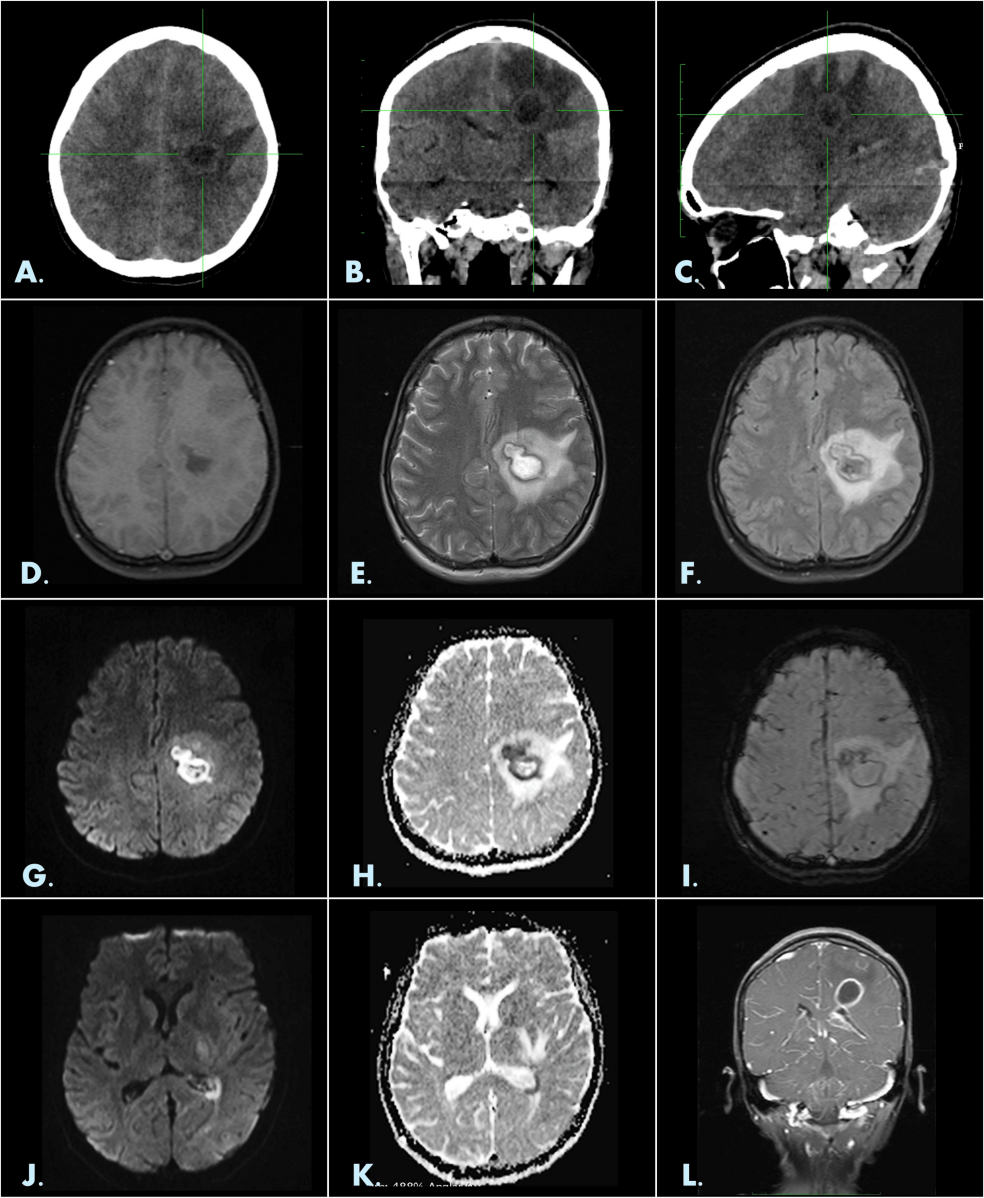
Imaging characteristics of the lesion in the left cerebral hemisphere: CT and MRI findings. Initial non-enhanced CT of the brain showing a 22 × 16 × 18 mm thin-walled lesion with central hypodensity in the left centrum semiovale and corona radiata, accompanied by perilesional edema. Axial (A), coronal (B), and sagittal (C) sections are displayed. MRI images reveal the lesion as hypointense on T1-weighted images (D), hyperintense on T2-weighted images (E), and heterogeneously hyperintense on FLAIR sequences (F). Diffusion restriction is shown (G and H). A dual rim sign is observed on susceptibility-weighted images (I). Additionally, diffusion restriction within the left lateral ventricle suggests rupture into the ventricle (J and K). Post-contrast MRI showed smooth, thin ring enhancement of the lesion with mild enhancement along the ependymal lining of the left lateral ventricle (L). CT, computed tomography; MRI, magnetic resonance imaging; FLAIR, fluid-attenuated inversion recovery
